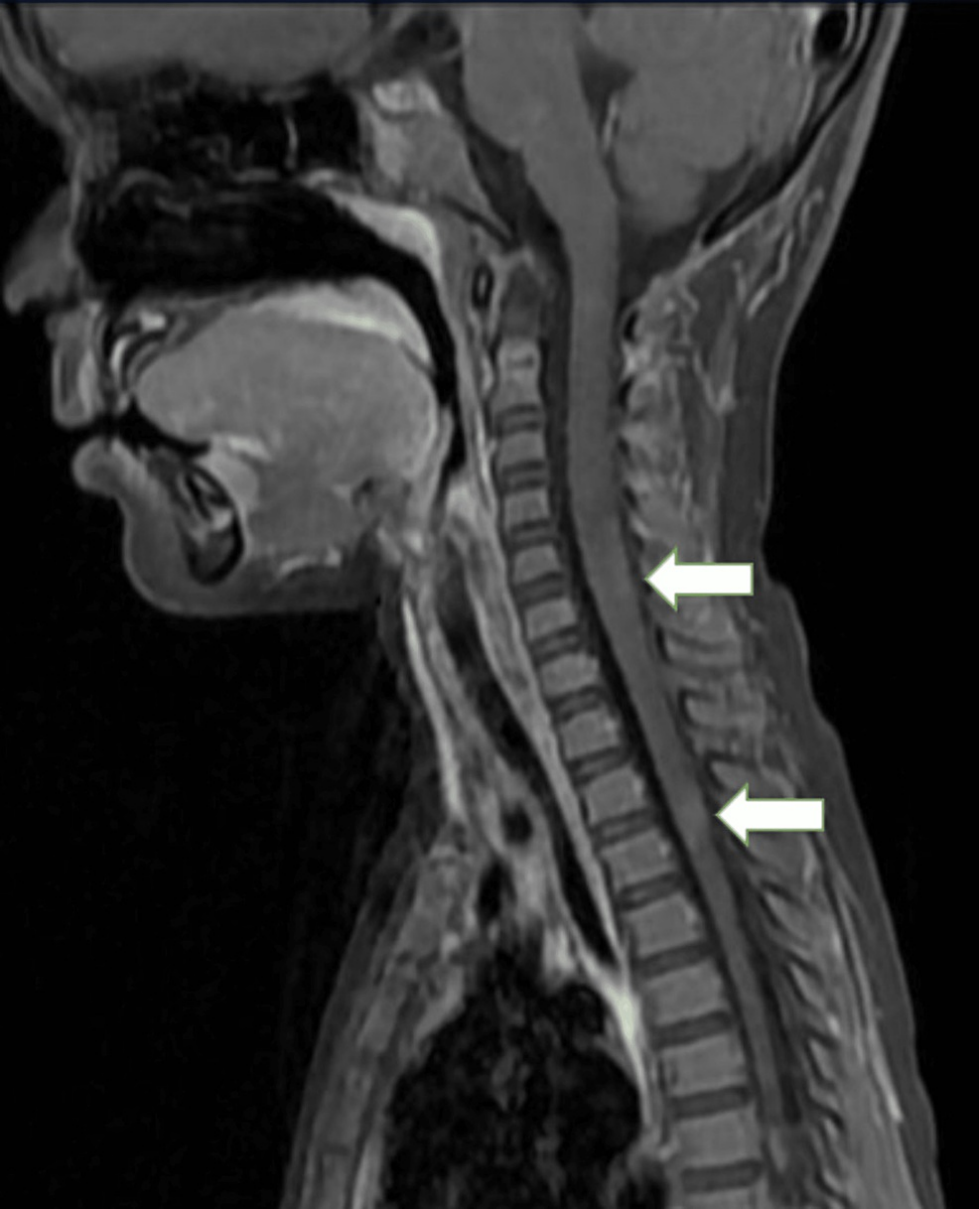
Saggital MRI showing lesions in the cervical and dorsal cord. MRI: magnetic resonance imaging
